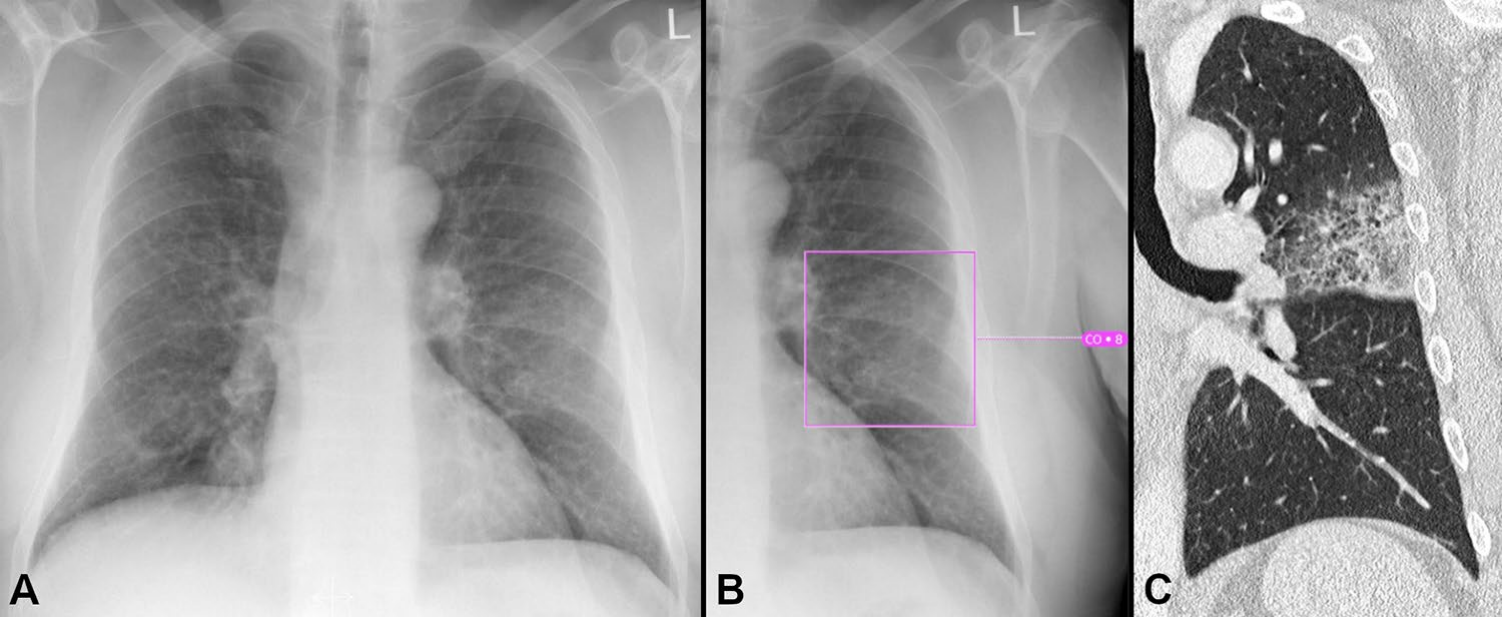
Example of a consolidation detected by the AI Rad Companion Chest X-ray (A, B) that was confirmed by a CT scan (C): 72 year old, male patient admitted to the hospital with fever, dyspnea and severe cough.
